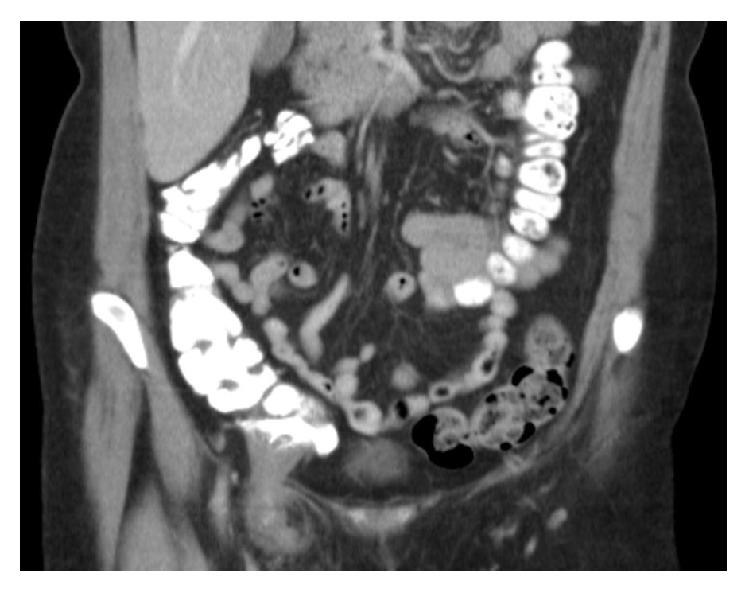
Preoperative abdominal CT image of the patient. Note the oral contrast in the colon, past the ileocaecal junction.
